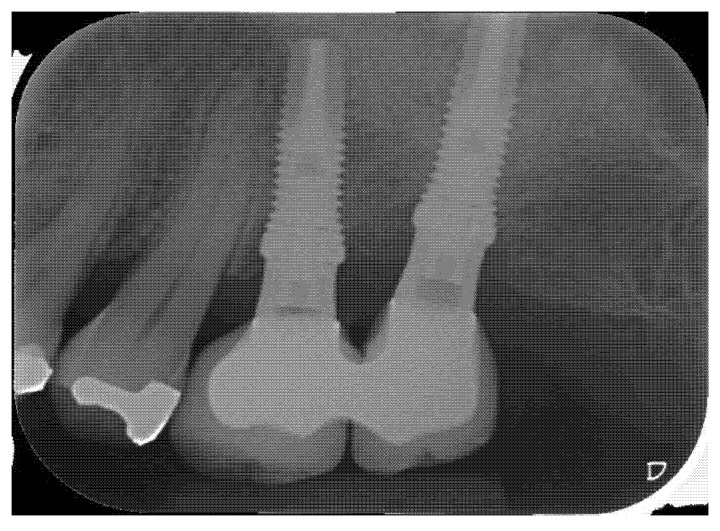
Radiographic control at 4 months.
